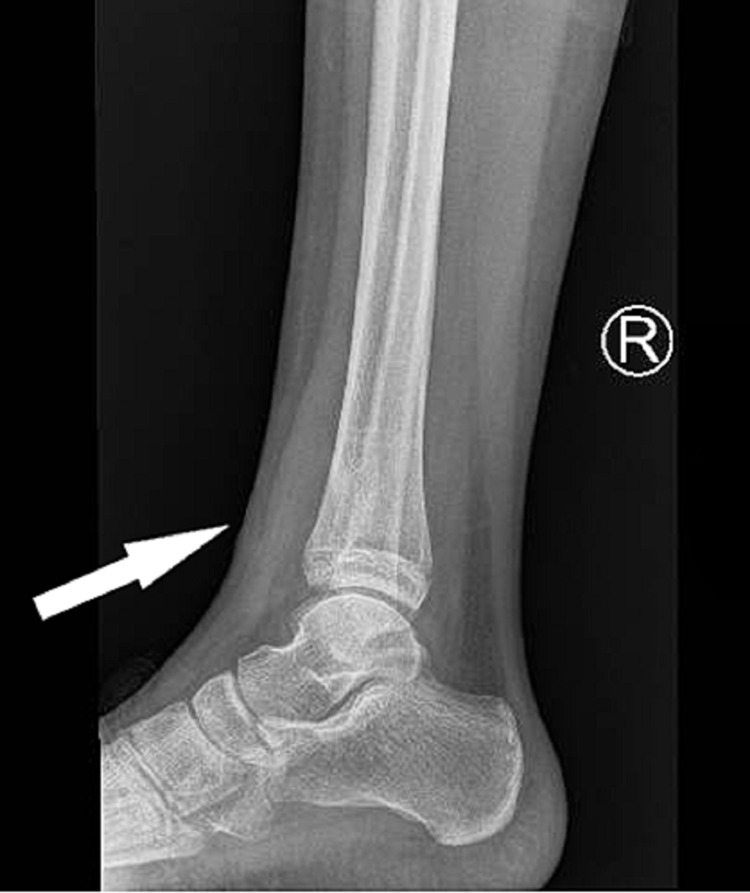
Post-operative X-ray showing good healing at the former site of Brodie's abscess
